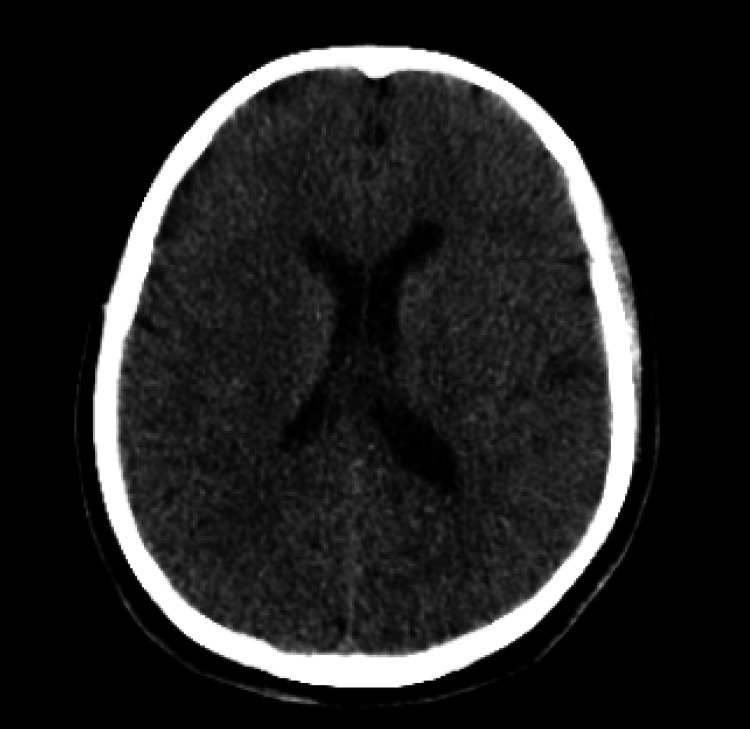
Normal brain CT CT: Computed tomography
